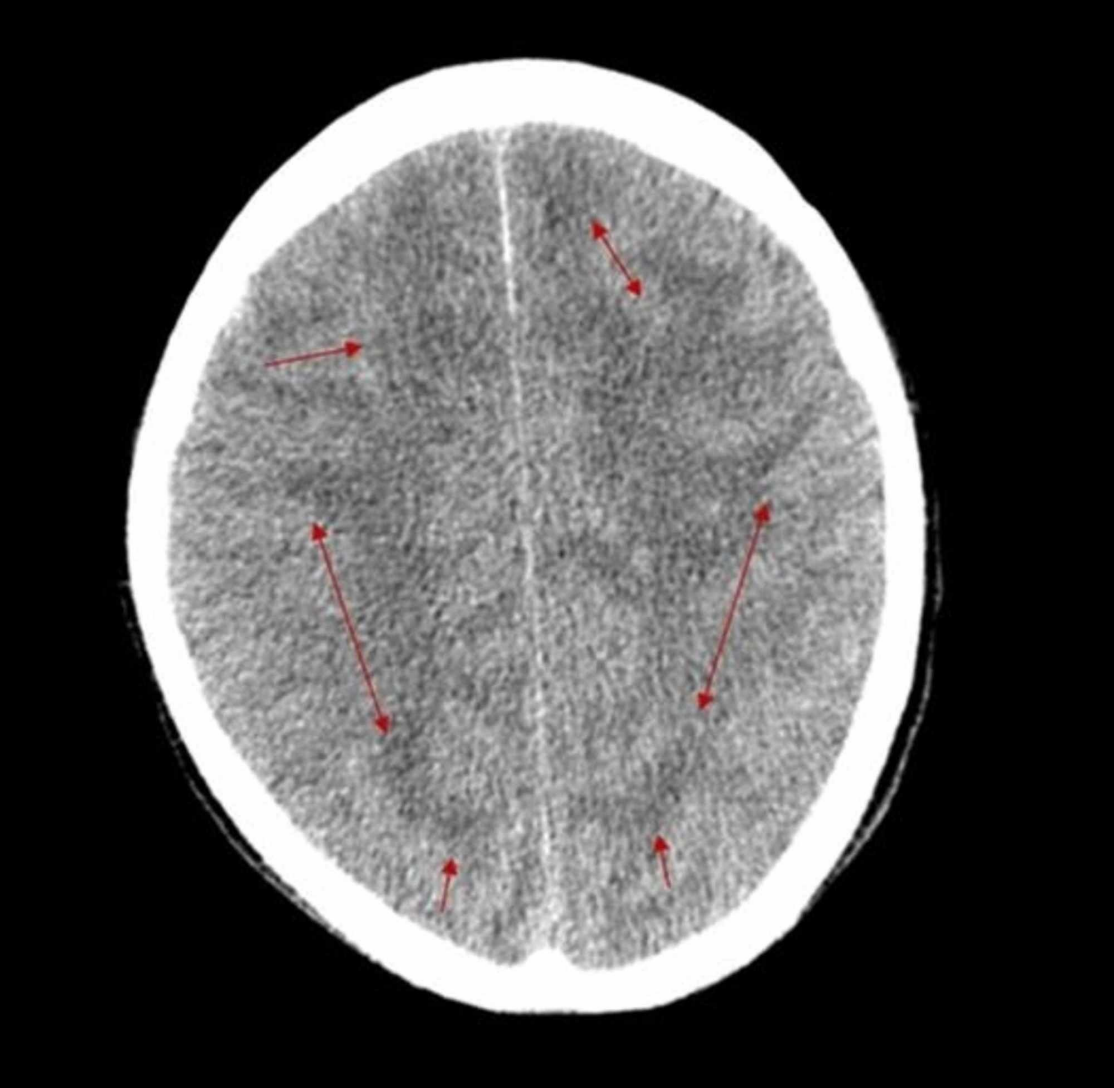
Axial image of non-contrast CT scan brain Extensive edema is visible in both cerebral hemispheres (red arrows). CT, computed tomography
